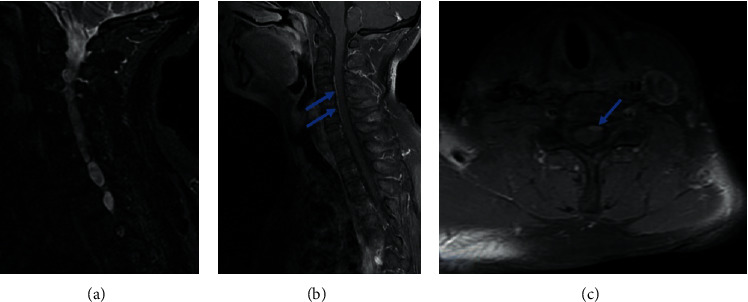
Cervical MRI, (a) STIR sagittal and (b, c) T1 sagittal and axial sequences after injection of Gadolinium; nodular contrast enhancement on the anterior surface of the left hemimarrow (blue arrows): appearance in favor of preganglionic lesions.
